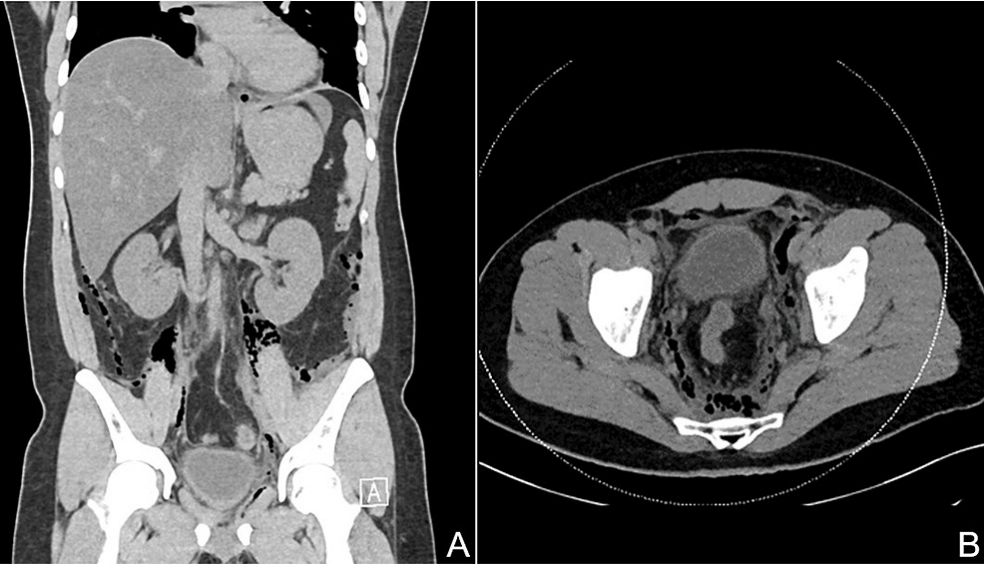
A non-enhanced CT scan of the abdomen and pelvis showing extensive emphysema of the extraperitoneal spaces. (A) A coronal image showing upward extension of retroperitoneal gas. (B) An axial section of the pelvis with widespread emphysema.
